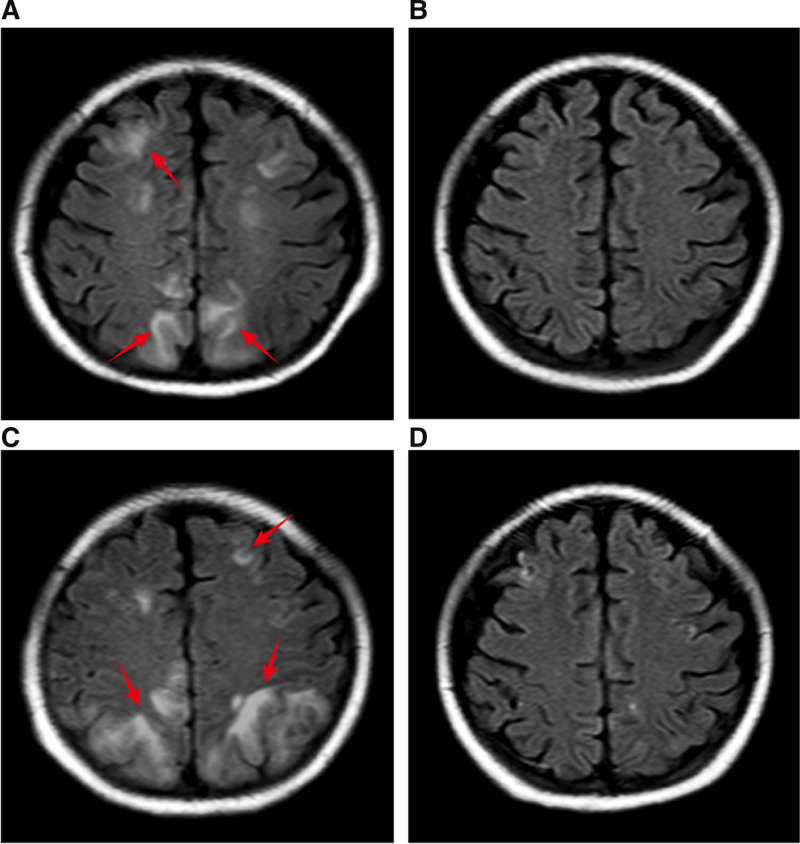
Seventeen-year-old female with 2 episodes of PRES. MRI during the first episode shows hyperintense lesions of the bilateral frontal and parieto-occipital (A). On a control MRI 2 wk later, the lesions have resolved completely (B). After 8 wk without any symptoms, the patient was admitted again because of a generalized seizure. MRI shows hyperintense lesions again that reveals the recurrence of PRES (C). With meticulous blood pressure control, reinforced hemodialysis and ultrafiltration, antiepileptic medication, and therapies to protect brain cells, repeat brain MRI showed significant improvement of PRES (D). MRI = magnetic resonance imaging, PRES = posterior reversible encephalopathy syndrome.
